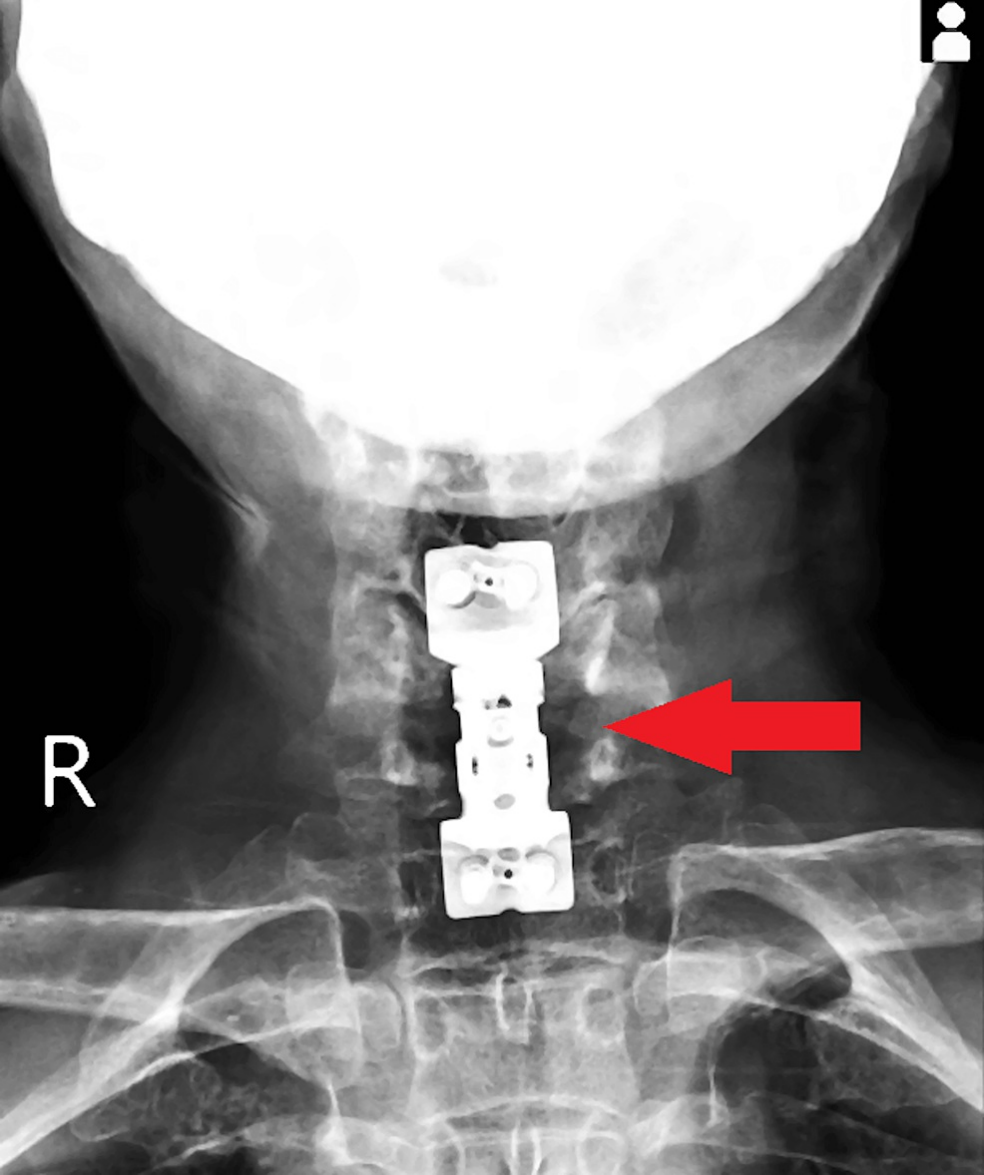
Postoperative X-ray of the patient
